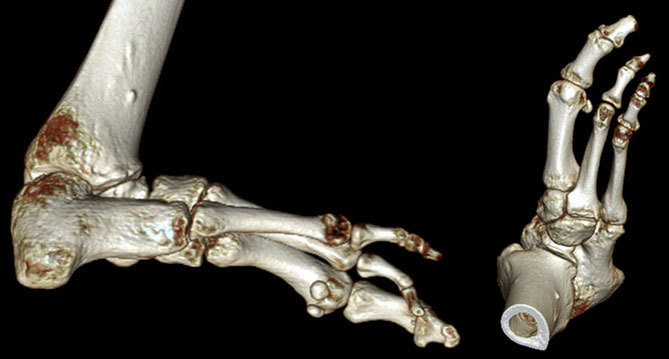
Three-dimensional reconstructed CT images of the right ankle and foot show club foot deformity, tarsal coalition and absent fourth and fifth rays.
